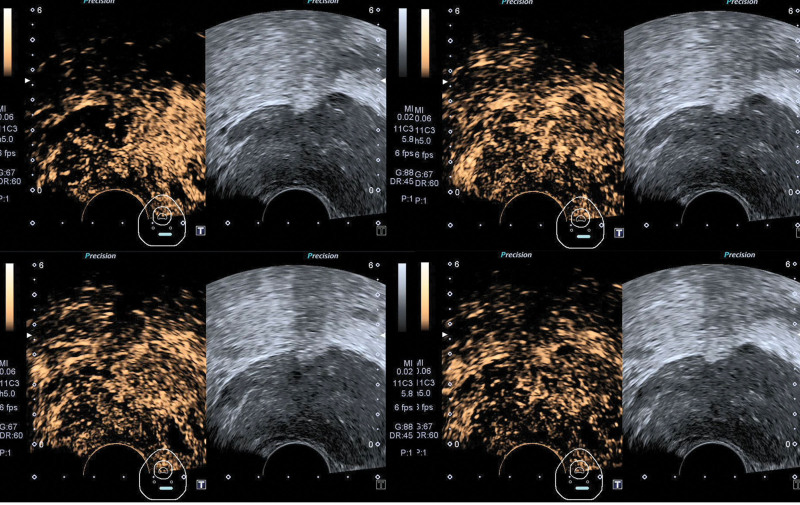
CETRUS presentation: (A) The left peripheral zone showed an inhomogeneous high enhancement pattern compared with the right side at the 18th. (B) The prostate is inhomogeneous after enhancement at the 29ths. (C and D) Ultrasound contrast agent in the whole prostate was fading simultaneously. (A)–(D) show the little region of the left peripheral zone (L1 and 2) without enhancement. CETRUS = contrast-enhanced transrectal ultrasound.
